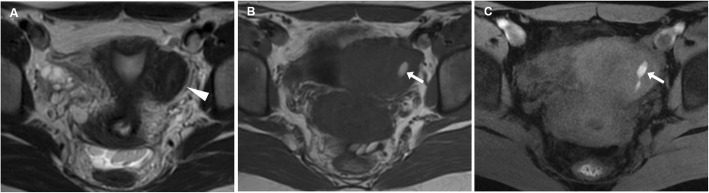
Leiomyoma with haemorrhagic degeneration in a 25-year-old woman suffering from acute pelvic pain. Oblique-coronal T2-weighted image (a) shows a subserosal leiomyoma, with predominant low signal intensity, arising from the left lateral wall of the uterus (arrowhead). On oblique-axial T1-weighted (b) and fat-suppressed T1-weighted (c) images, the mass demonstrates a central region of hyperintense signal (arrows) corresponding to haemorrhagic degeneration
